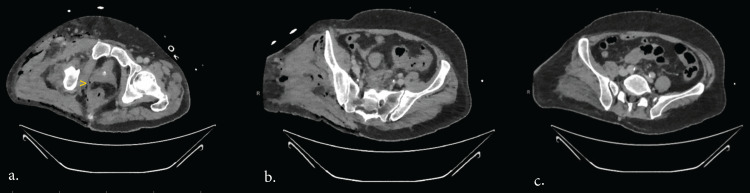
Cross-sectional imaging showing air below the peritoneal reflection Postoperative CT scan revealed the following: (image a) gas around the rectum (yellow arrow) caudally, below the peritoneal reflection. Sigmoid diverticula apparent (image b) without any pneumoperitoneum in more cephalic cross sections (image c).
